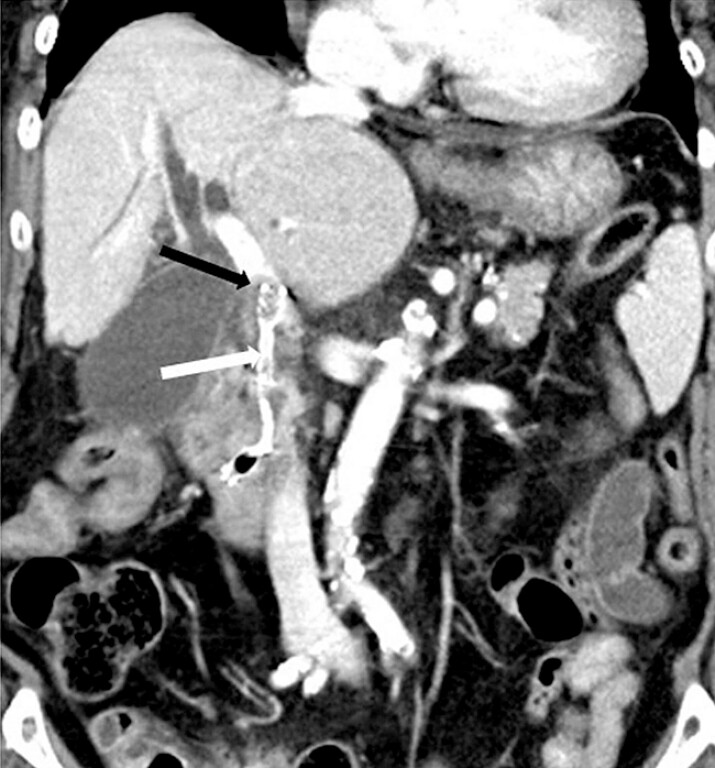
Contrast-enhanced computed tomography obtained 2 hours post-endoscopic retrograde cholangiopancreatography showing maldeployed fully covered self-expandable metal stent (white arrow) in the portal vein via the distal bile duct mass, with associated portal vein thrombosis (black arrow).
